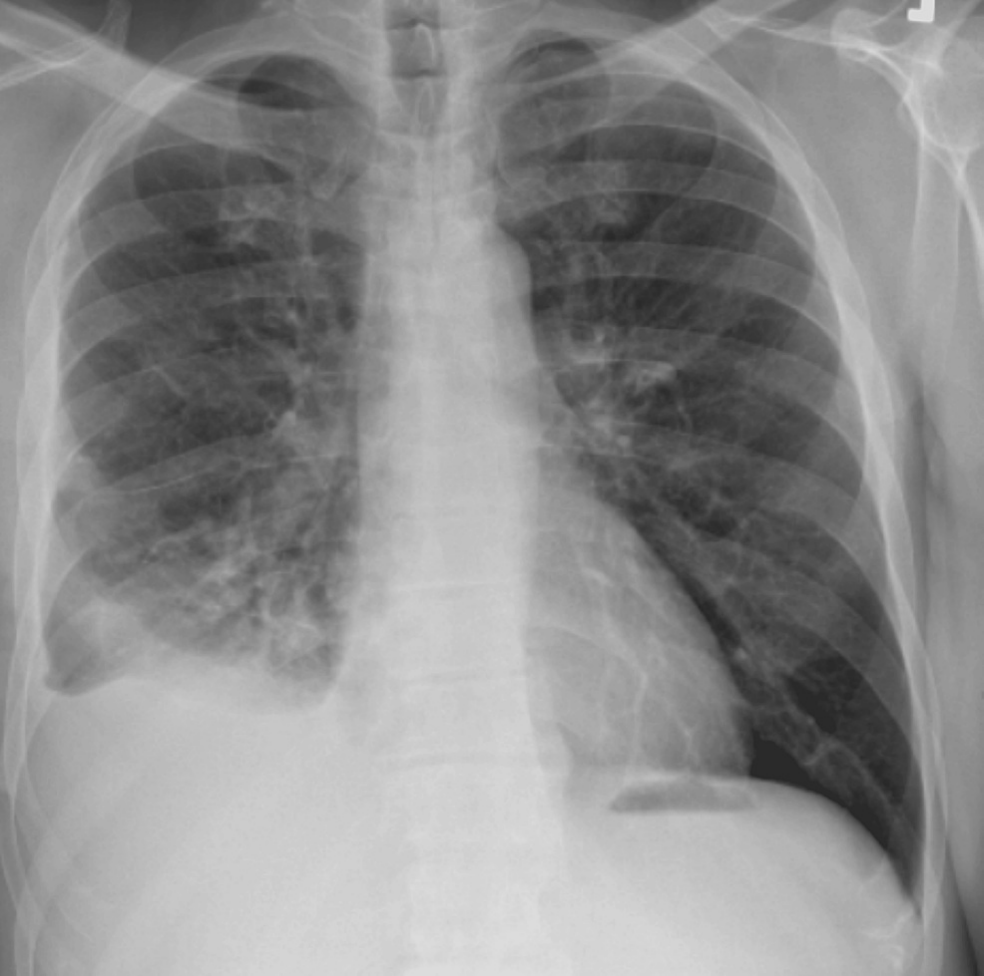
Chest X-ray showing significant interval improvement in aeration in the right lower lung parenchyma, mild right-sided pleural effusion still present tracking along the lateral wall.
